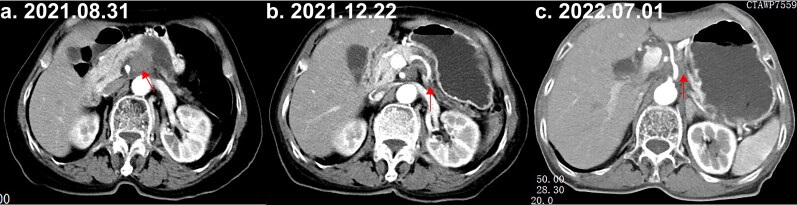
Abdominal CT showing pancreatic cancer post-chemotherapy and immunotherapy. The red arrow highlights the tumor locations. (a) Initial abdominal CT scan at admission. (b) Abdominal CT scan after AG and camrelizumab treatment. (c) Abdominal CT scan following completion of the second chemotherapy course with FOLFIRINOX.
